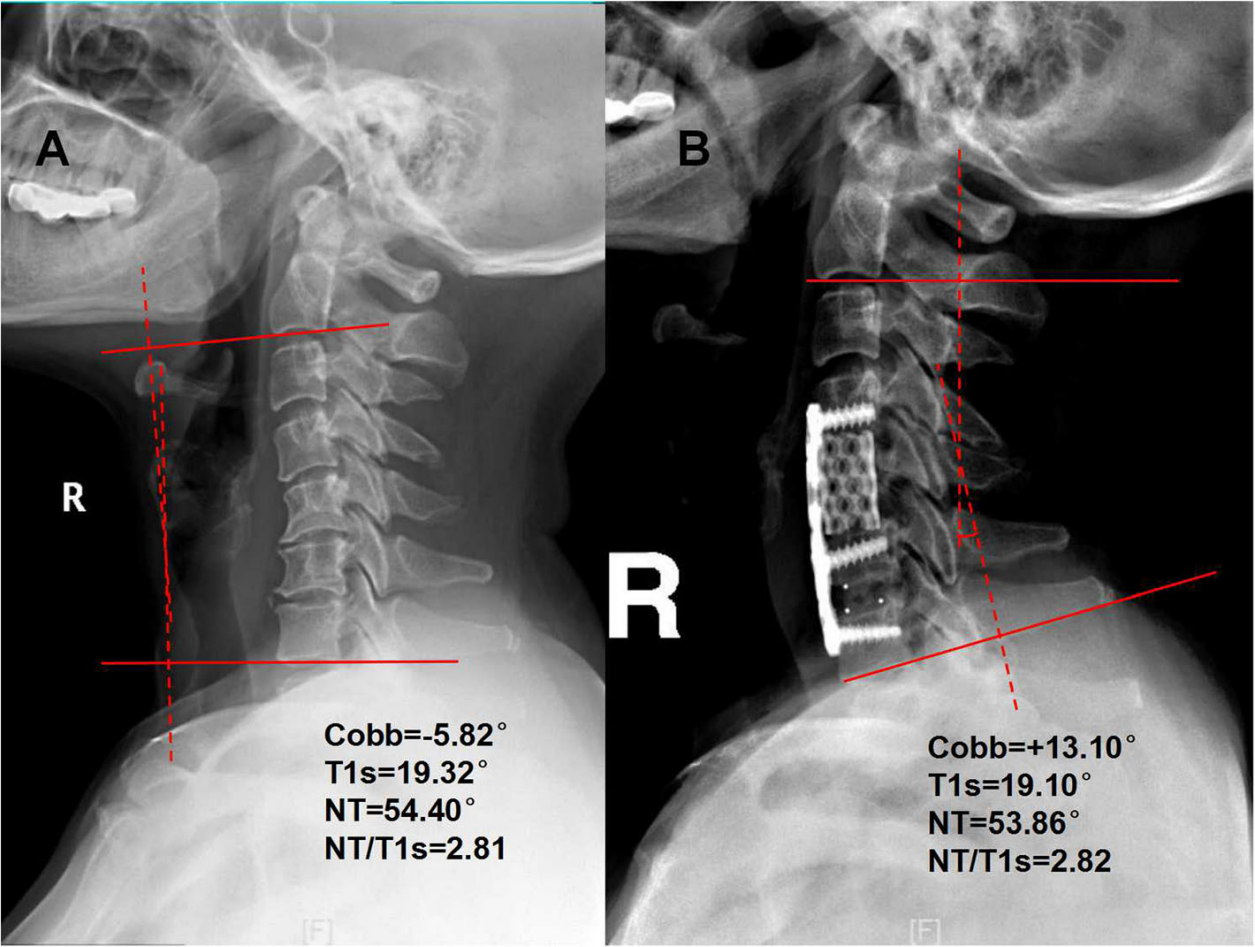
A case (preoperative and postoperative X-ray films). T1s, T1 slope; NT, neck tilt
